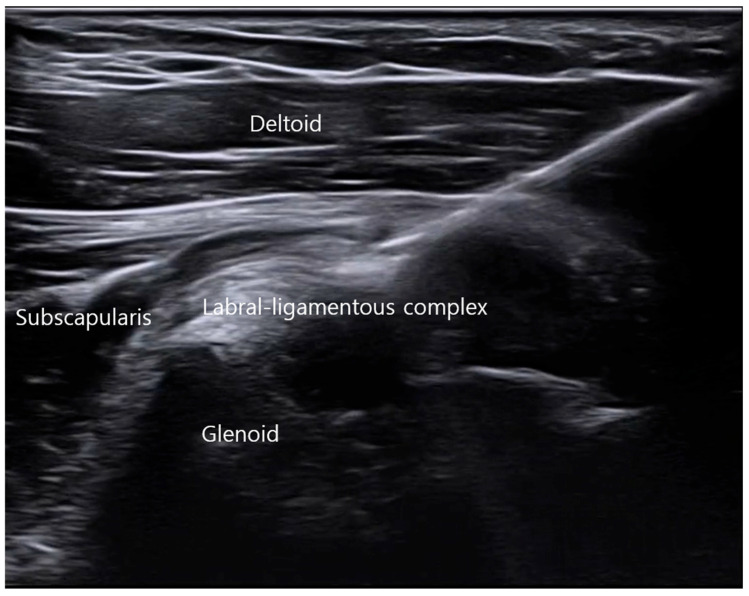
Ultrasound-guided in-plane needle approach targeting the anterior labral–ligamentous complex.
